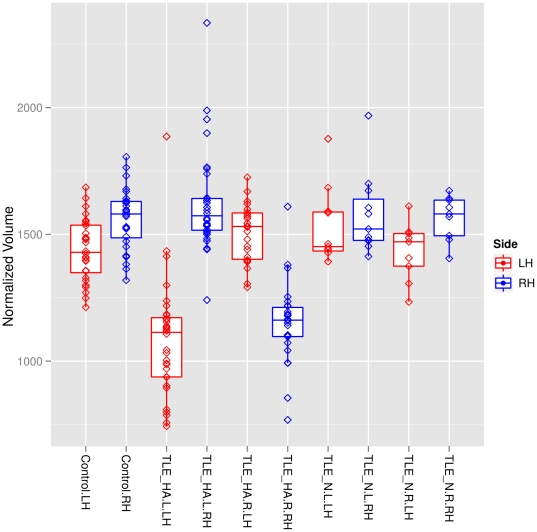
Hippocampal volumes in patients and controls. Horizontal lines show the medians, boxes indicate interquartile ranges, whiskers show the minimal and maximal values inside the main data, and lozenges show individual values. Blue, right hippocampi; red, left hippocampi. TLE-HA, TLE with hippocampal atrophy; TLE-N; TLE with normal MRI on visual inspection. Suffixes _L and _R denote left and right sided seizure focus, respectively.
